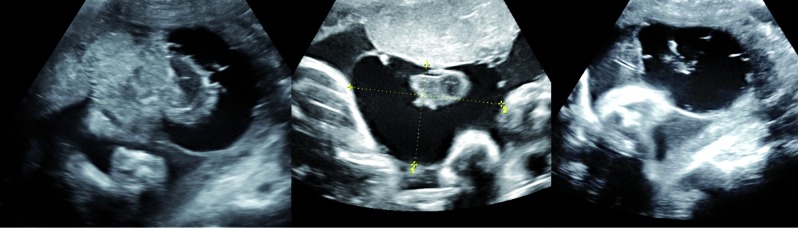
Ultrasound at 32 weeks with multiple large (5cm) cysts with intracystic hemorraghe.
